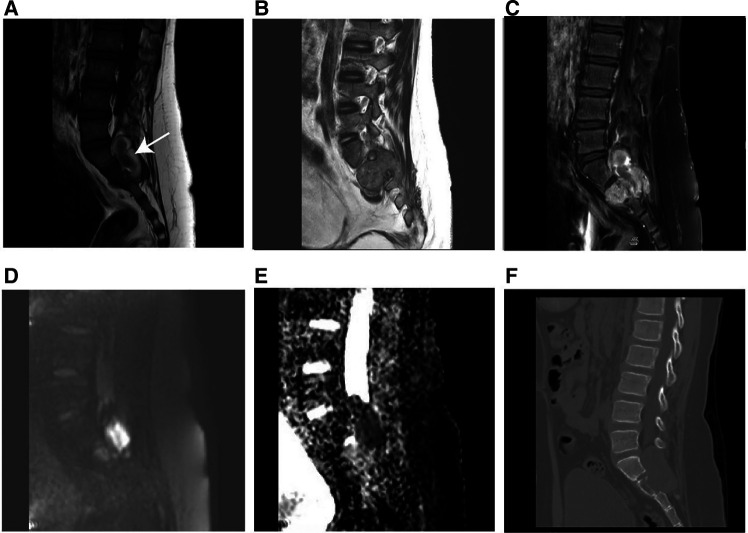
MR imaging results of soft tissue RT in a male of 90 months (clinical manifestations: sacrococcygeal paroxysmal pain for 1.5 years, gradually aggravated). (A) T1-weighted MR imaging indicated a moderately hypointense mass with hemorrhage (arrow). (B) T2­weighted MR imaging showed a moderately hyperintense mass extending from the intervertebral foramen to the posterior right pelvic wall. (C) T1-weighted enhanced MR imaging revealed heterogeneous enhancement. (D) Hyperintensity on the DWI image. (E) The mean apparent diffusion coefficient (ADC) value of the mass was 0.722 × 10^−3^ mm^2^/s. (F) The sagittal CT image revealed vertebral bone destruction.
